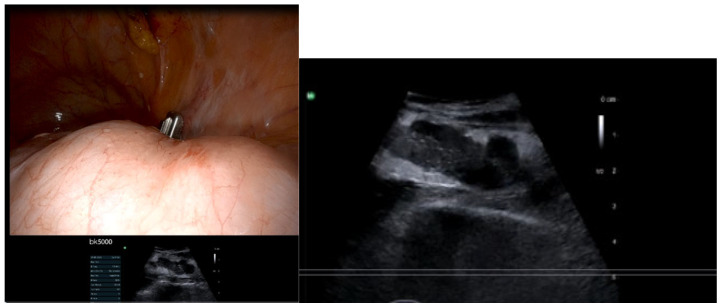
Identifying an enlarged lymph node. In the ultrasound scan image at the bottom of the picture, you can see the hypoechogenic large lymph node medial to the right external iliac vein (courtesy of A. Sayasneh).
